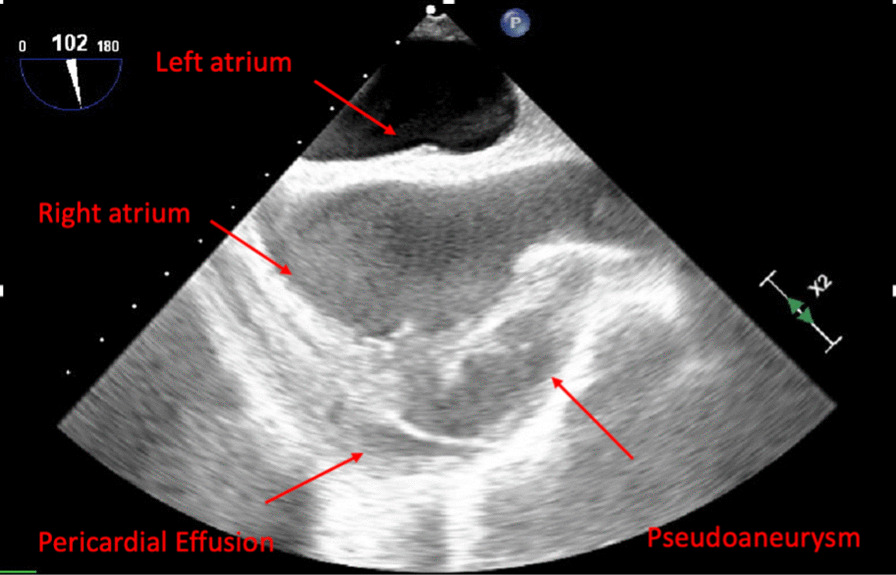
Transesophageal echocardiogram of pseudoaneurysm. Pseudoaneurysm with pericardial effusion redemonstrated on transesophageal echocardiogram arising from right atrium
